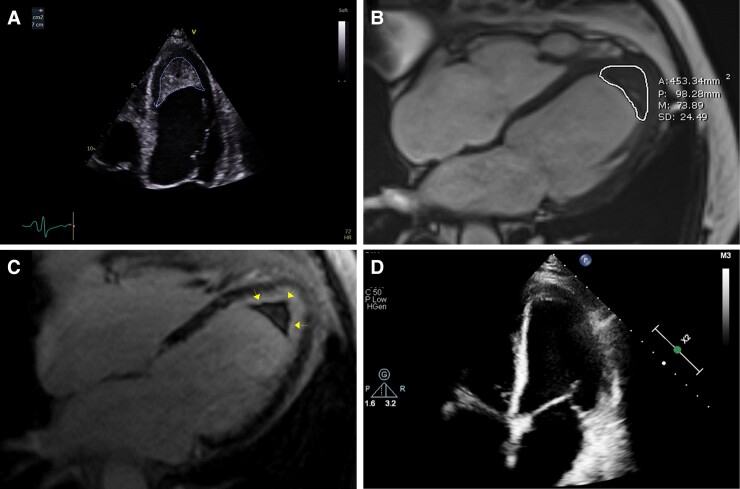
Pre-treatment: (*A*) B-mode echocardiography demonstrating apical LV mass. Left-ventricular ejection fraction is 33%, left-ventricular internal diameter end diastole 50 mm, and left-ventricular internal diameter end systole 41 mm. (*B*) Balanced steady-state precession cine MRI in four-chamber view demonstrating LV mass. (*C*) Late gadolinium enhancement confirms that it is homogenous hypointense mass in LV consistent with LV thrombus. Also, endomyocardial pattern of fibrosis of the left ventricle due to inflammation can be seen (yellow arrows). Post-treatment: (*D*) Repeat echocardiography after 9 months time confirms resolution of majority of the thrombus with now potentially less dangerous established transmural thrombus layer only. Left-ventricular ejection fraction is 37%, left-ventricular internal diameter end diastole 52 mm, and left-ventricular internal diameter end systole 44 mm.
